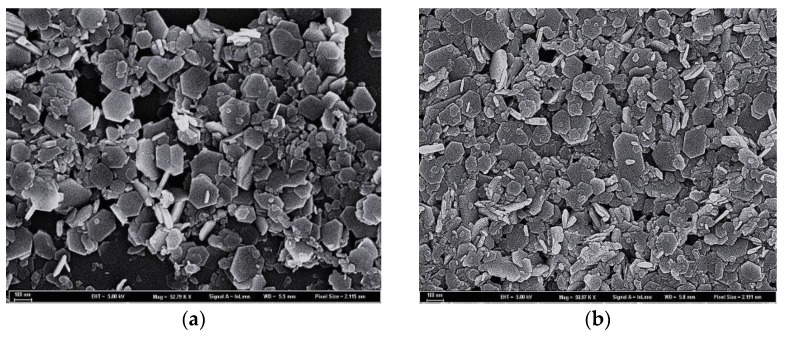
SEM images of the products of hydration in the ultrasound field at 120 min: (a) Δn_Cl_^−^ = 21.5 mol/kg; (b) Δn_Cl_^−^ = 7.5 mol/kg.
